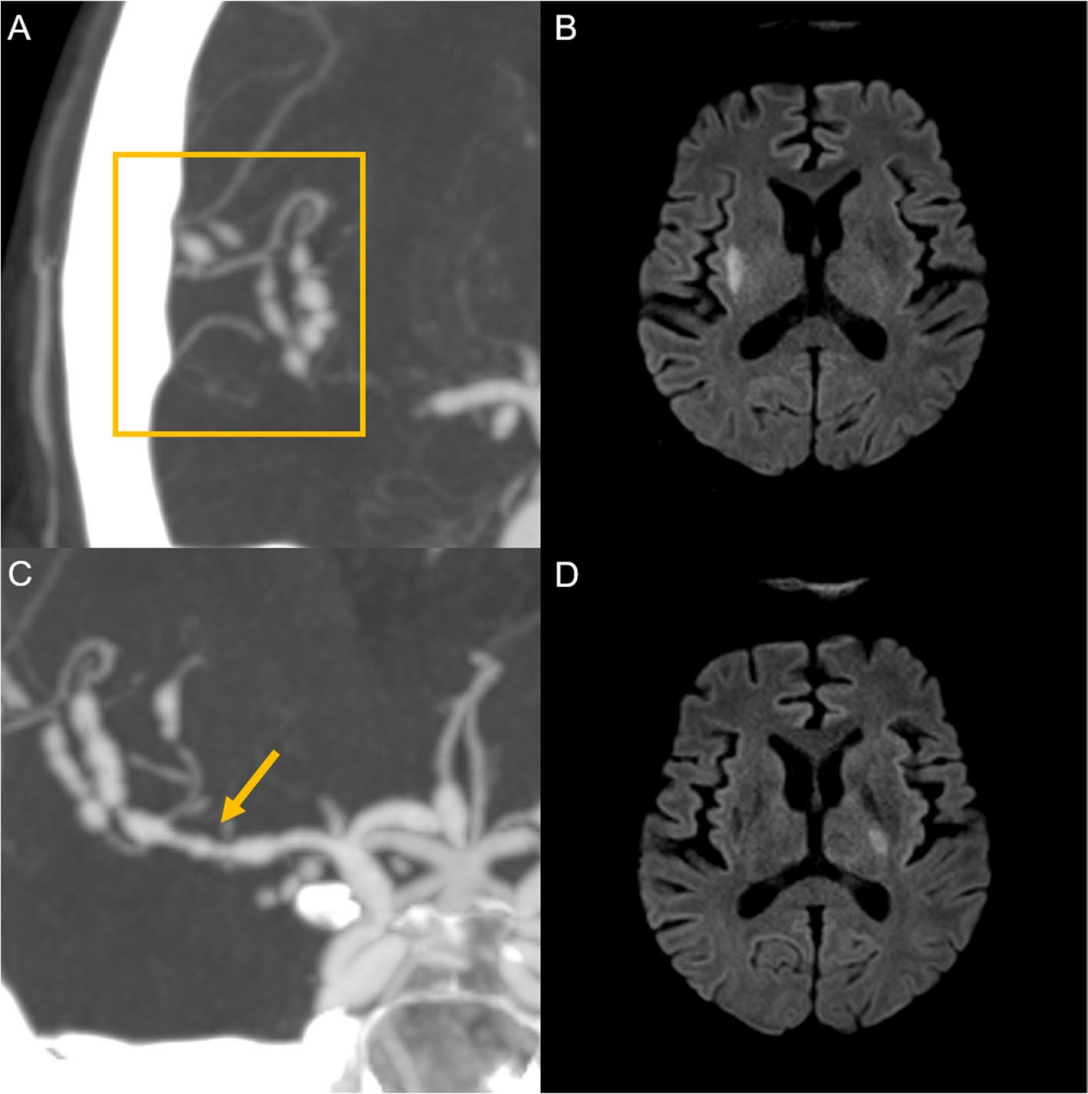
Neuroimaging findings at initial presentation. A Initial coronal computed tomography angiography (CTA) showing multifocal “string of bead” appearance of the intracranial arteries (yellow box), which was more pronounced in the right hemisphere. B Initial diffusion-weighted magnetic resonance imaging (MRI, b = 1000) showing an acute focal infarction in the right corona radiata. C Follow-up coronal CTA reveals newly developed arterial stenosis and dilatation involving the M1 segment of the right middle cerebral artery, indicating disease progression. D Follow-up diffusion-weighted MRI (b = 1000) revealing a newly developed acute infarction in the left posterior limb of the internal capsule
